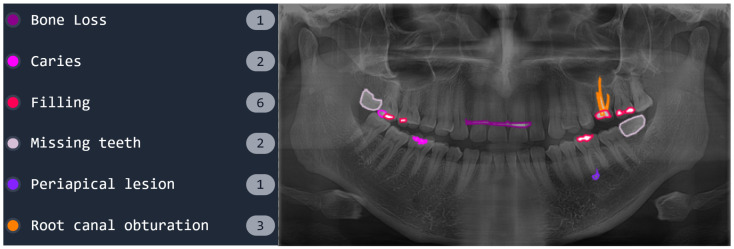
Sample panoramic radiograph of a patient with multiple dental conditions.
